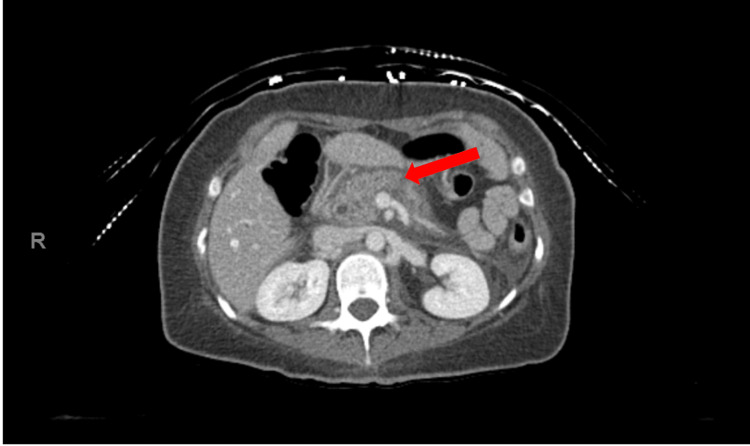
Abdominal and pelvic computed tomography (CT) showing interstitial pancreatitis (red arrow).
